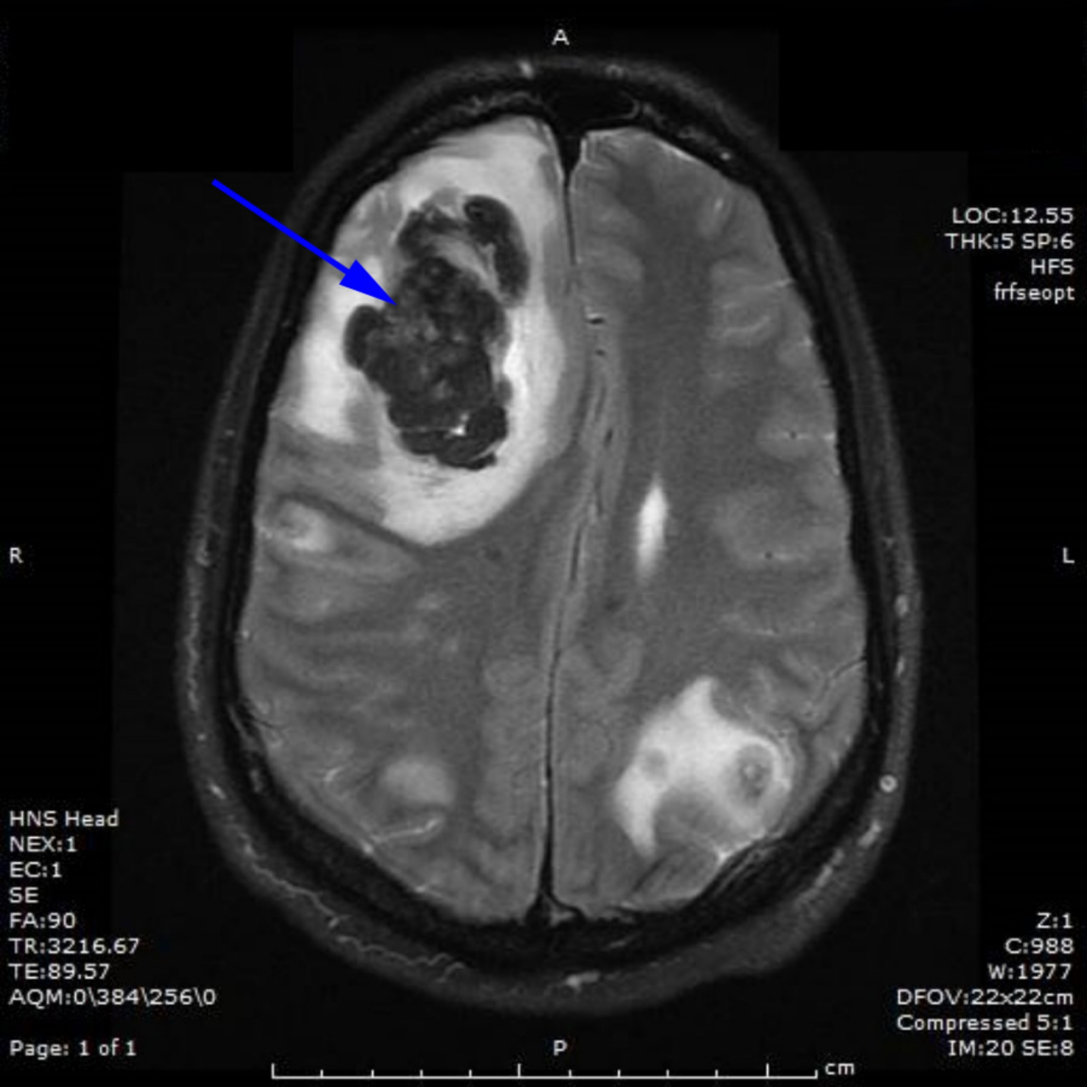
Brain magnetic resonance imaging (MRI) MRI of the brain with 5.5 x 4 cm hemorrhagic mass and midline shift.
